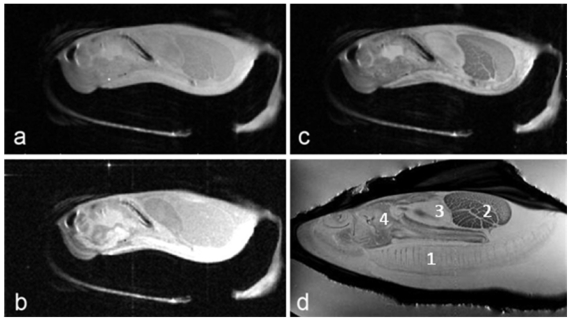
(a) Proton density (b) T1- and (c) T2-weighted images of an Eastern oyster (*Crassostrea virginica*). (d) T2-weighted image of another oyster in a life support system. 1: gills, 2: adductor muscle and hemolymph sinuses, 3: heart, 4: stomach. Note the fine anatomical structure displayed in the image with the gills fully extended.
